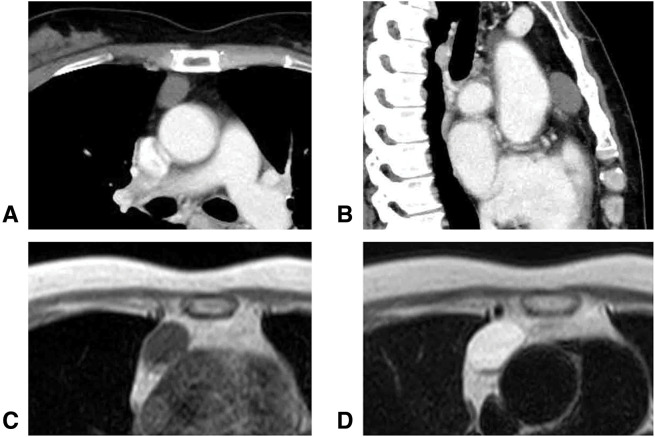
Preoperative imaging. Chest CT shows a 28 × 15-mm round nodule in the anterior mediastinum (A, B). MRI shows the nodule as low intensity on T1-weighted imaging (C) and high intensity on T2-weighted imaging (D).
